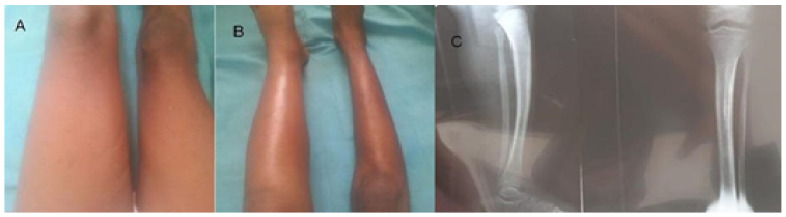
(A) Patient on initial presentation, left thigh swelling (mid-thigh circumference 31 cm). Right thigh (mid-thigh circumference 25 cm); (B) Patient on initial presentation, left leg swelling (mid-leg circumference 26 cm). right mid-leg circumference 20.5 cm and (C) Patient on initial presentation, left and right leg X-ray.
